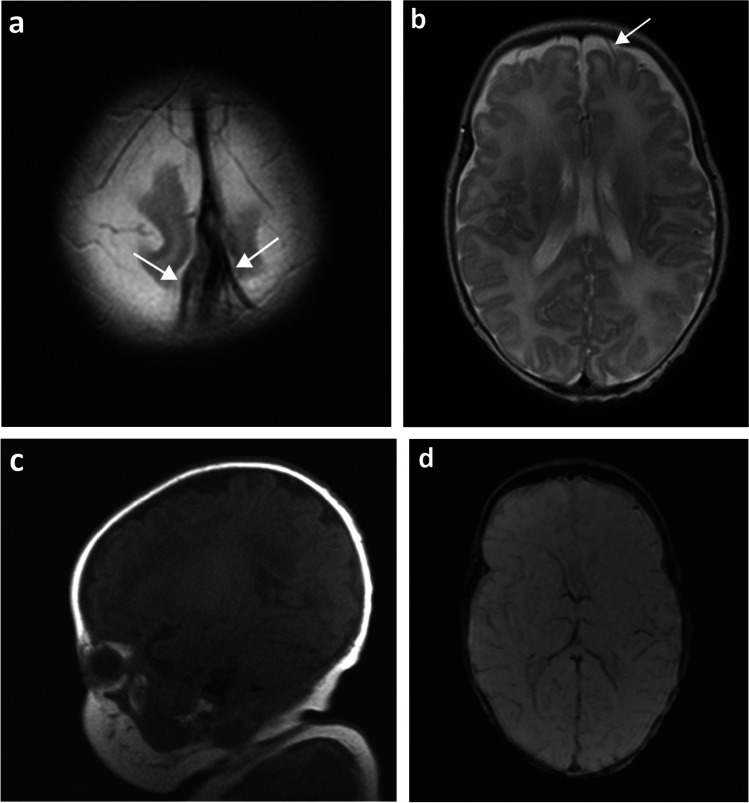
A 16-week-old boy born extremely premature at 25 gestational weeks underwent magnetic resonance imaging at term-equivalent age to assess for subdural haemorrhage. a Axial T2-weighted image at the upper cerebral convexity shows bridging veins (*arrows*) located in the extra-axial compartment, draining into the superior sagittal sinus. b Axial T2-weighted image at the level of the lateral ventricles shows the vessel (*arrow*) traversing the subarachnoid space, confirming that this compartment is the subarachnoid space rather than a subdural collection. c Sagittal T1-weigthed image shows no hypo-, iso-, or hyperintense collections relative to adjacent cortex within the subdural space, suggesting the absence of subdural haemorrhage. d Axial susceptibility-weighted image shows no hypointensities in the subdural space, suggesting the absence of subdural haemorrhage
